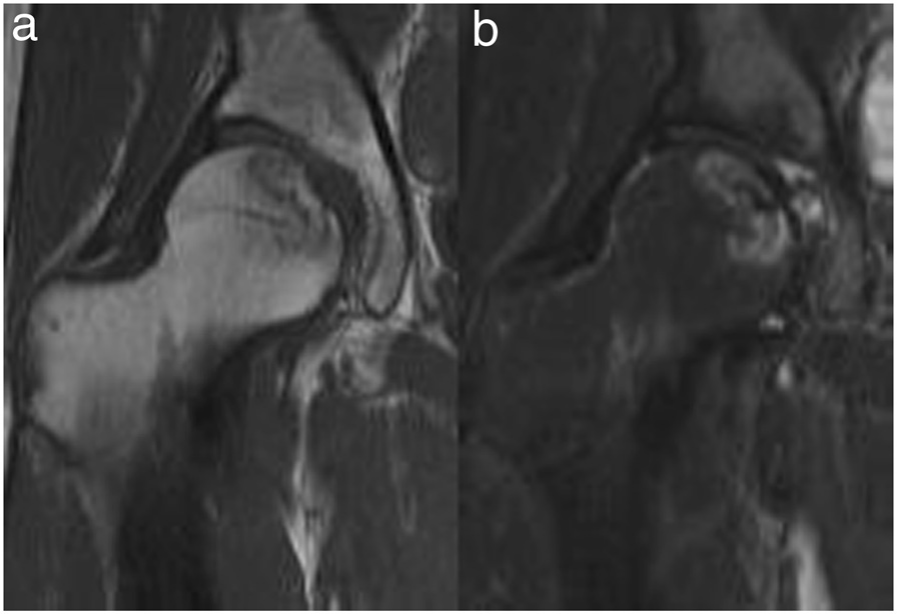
Preoperative magnetic resonance imaging. a. T1-weighted imaging: a band sign characteristic of idiopathic osteonecrosis of the femoral head was observed. b. T2 short-tau inversion recovery: a high intensity was noted in the acetabular labrum, but no bone marrow edema or hydrarthrosis suggesting collapse was present in the necrotic region of the femoral head.
